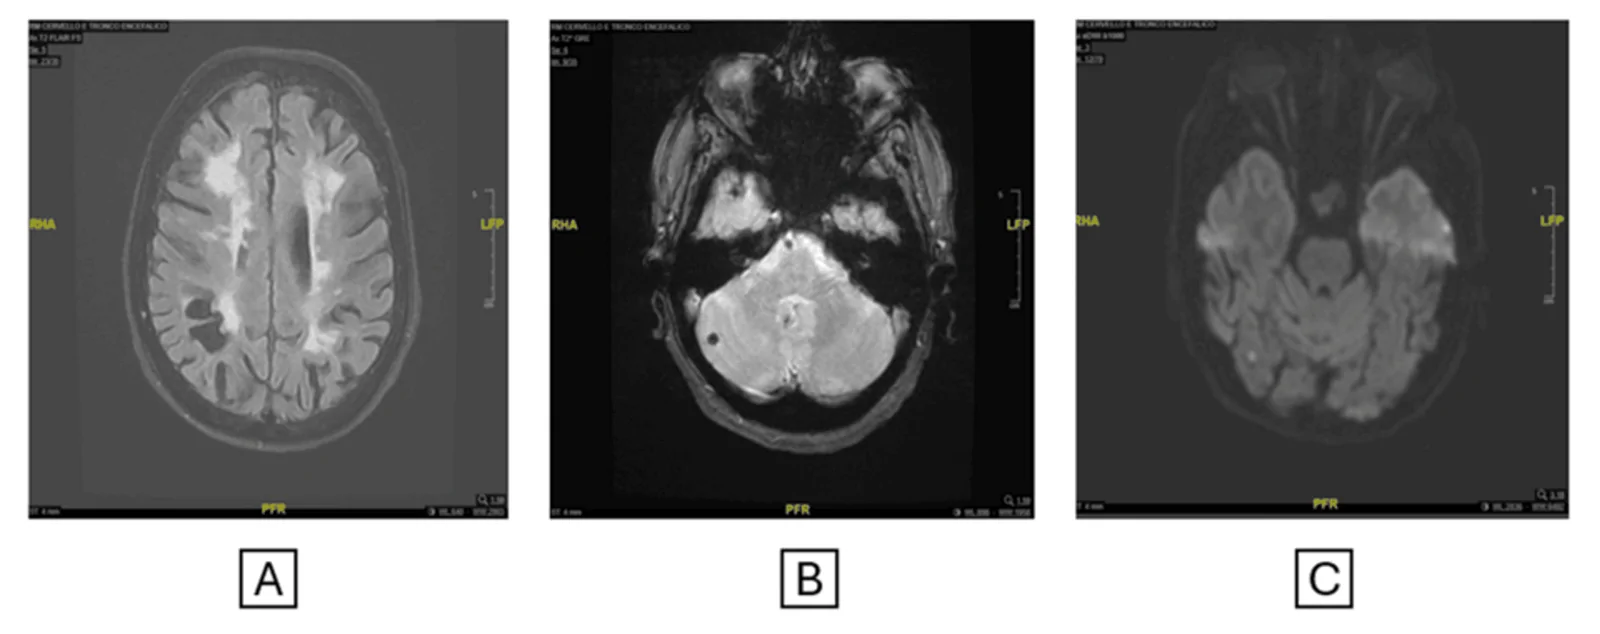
Brain MRI showing multifocal ischemic infarcts. (A) Axial fluid-attenuated inversion recovery (FLAIR) image demonstrating marked chronic ischemic white matter changes. (B) Axial T2-weighted image demonstrating prior hemorrhagic changes. (C) Diffusion-weighted imaging (DWI) demonstrating multiple acute infarcts involving the right frontal subcortical region, vertex, left posterior parietal lobe, and right occipital lobe.
